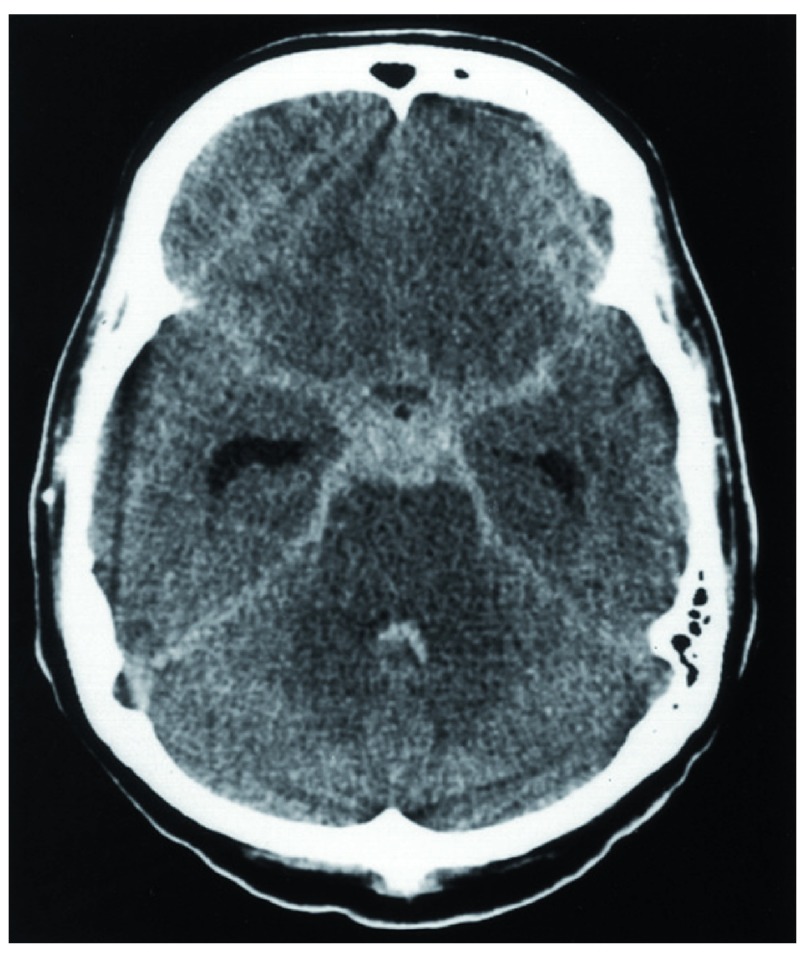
CT image of subarachnoid haemorrhage. Non-contrast CT scan of brain showing subarachnoid haemorrhage in classical “star sign” distribution with blood distributed along basal vessels.
